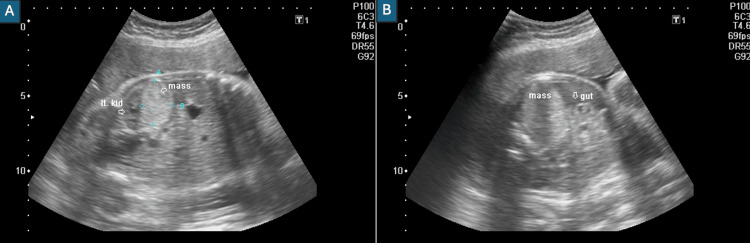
Prenatal ultrasonography demonstrating the echogenic lobulated space-occupying lesion in the fetal suprarenal region. (A) Echogenic mass displacing the left kidney. (B) Relationship of the mass with the fetal gut.
